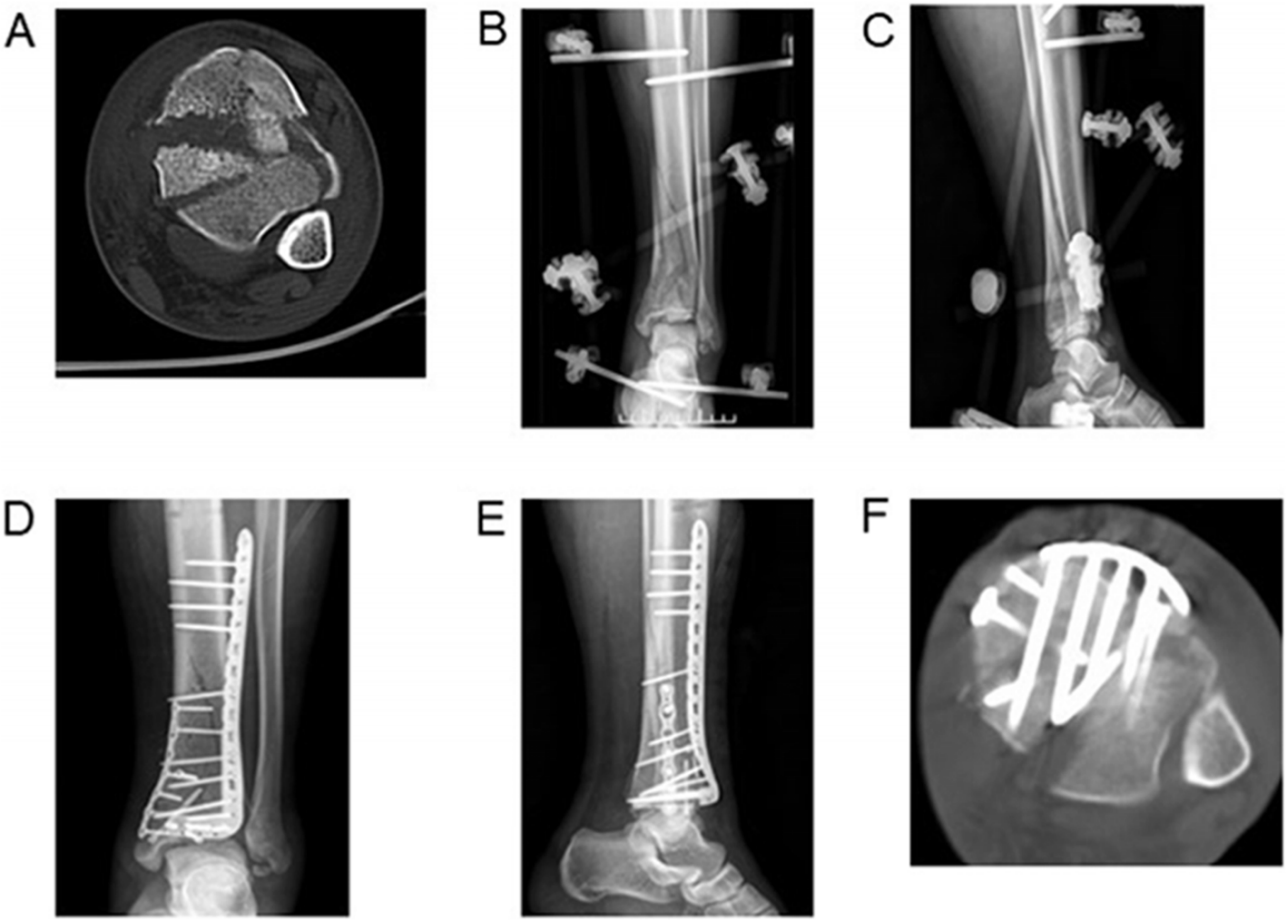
A 20-year-old man involved in a fall developed AO/OTA C3 pilon fracture. A) Preoperative transverse computed tomography (CT) scans showed severe fracture displacement of the distal tibial articular surface. B & C) The anteroposterior and lateral view of the ankle showed that external fixation was used to maintain the length and axial alignment of the limb after undergoing external fixation in the first stage for 19 days. D & E) The lateral view showed good reduction and fixation after undergoing open reduction and internal fixation through the simple anterior approach in the delayed procedure. F) Postoperative transverse CT scans showed articular surface step-off of more than 5 mm
